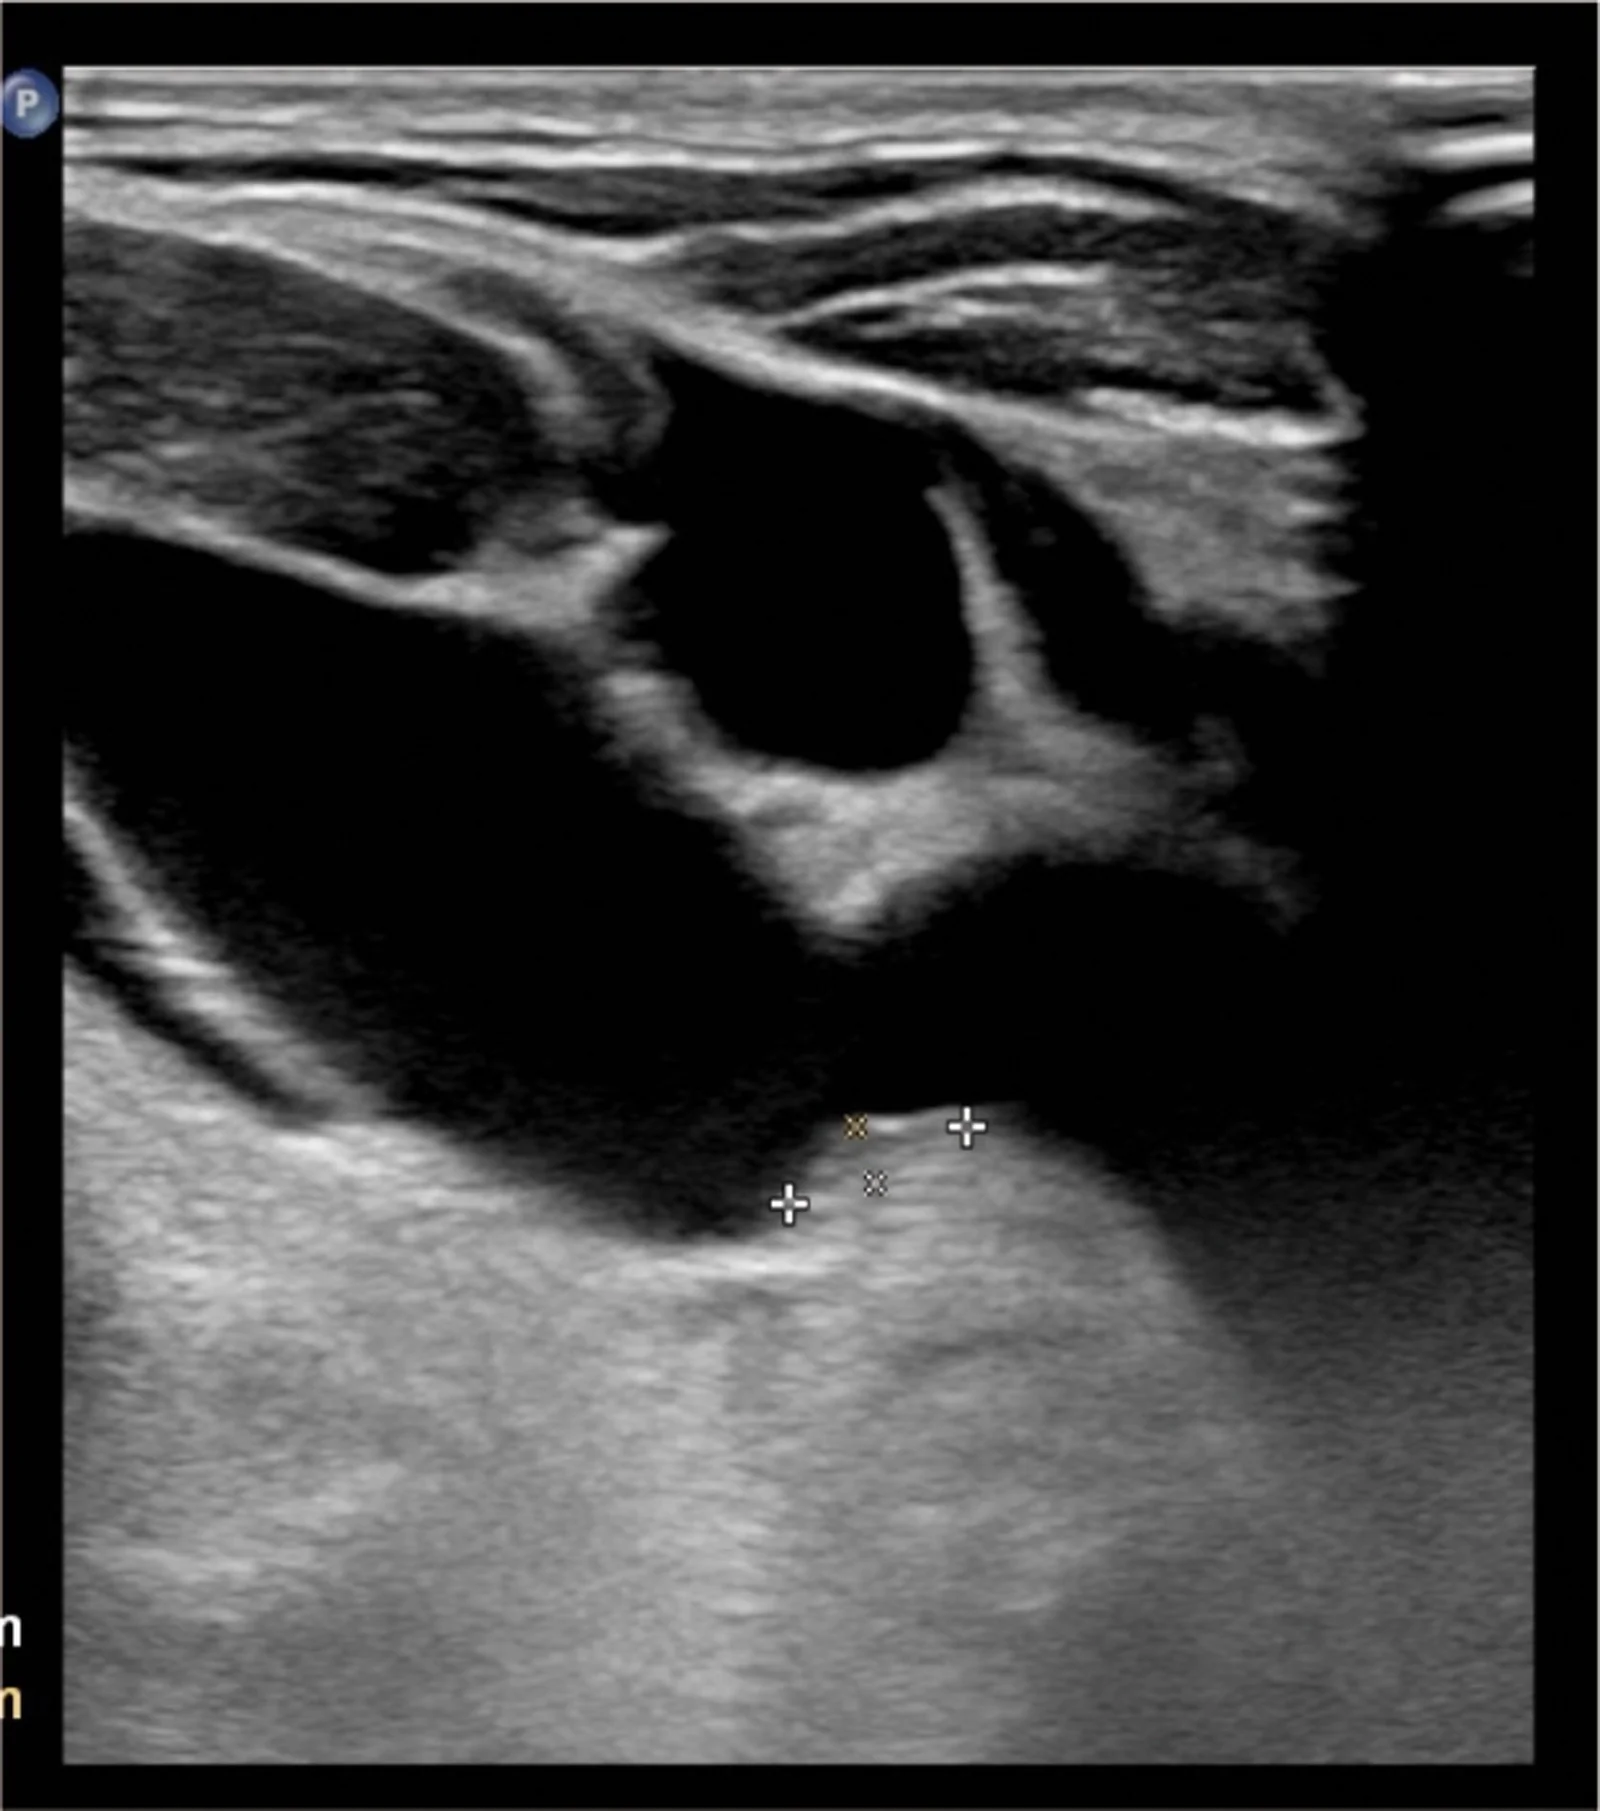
Vascular ultrasound. The cross indicates the atheromatous plaque, which measured 4.8 × 1.4 mm at the time of examination.
